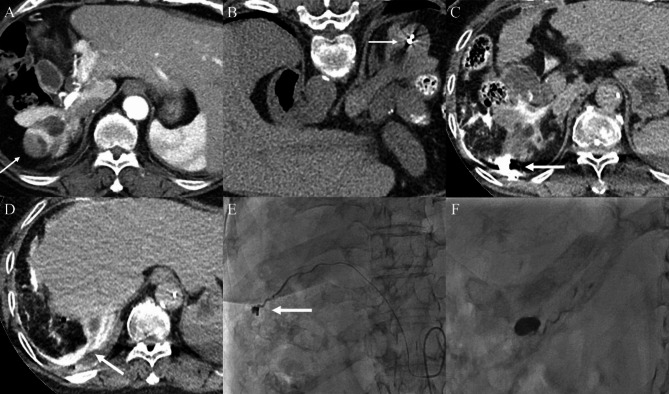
A case of a 66-year-old female having bleeding after microwave ablation (MWA). (A) Pre-procedure liver dynamic computed tomography (CT) revealed hepatocellular carcinoma (HCC) in the segment VII subcapsular area (arrow). (B) After microwave antenna placement (arrow), non-contrast CT was performed to determine if the lesion was appropriately targeted. (C, D) After completion of ablation, post-ablation three-phase dynamic contrast-enhanced CT was performed, and contrast extravasation was observed (arrows). (E, F) On transarterial angiography, active bleeding from the right inferior phrenic artery was noted (arrow); thus, embolization with a mixture of NBCA and Lipiodol was performed
